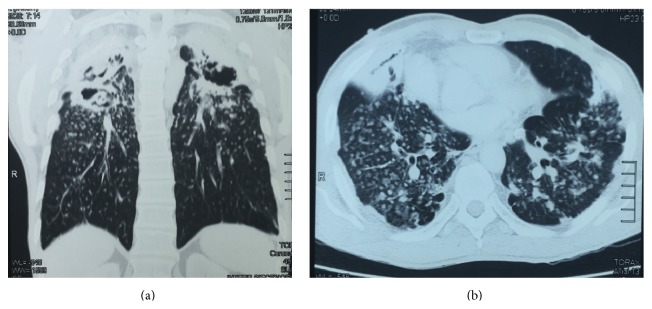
Thorax computed tomography: large pulmonary opacities in the upper lobes of both lungs, air bronchograms and calcifications, bilateral diffuse nodular infiltrates with areas of pleural thickening, nodular interstitial infiltrate associated with ground glass opacities, and cavitations with thick walls. (a) Coronal plane. (b) Axial plane.
